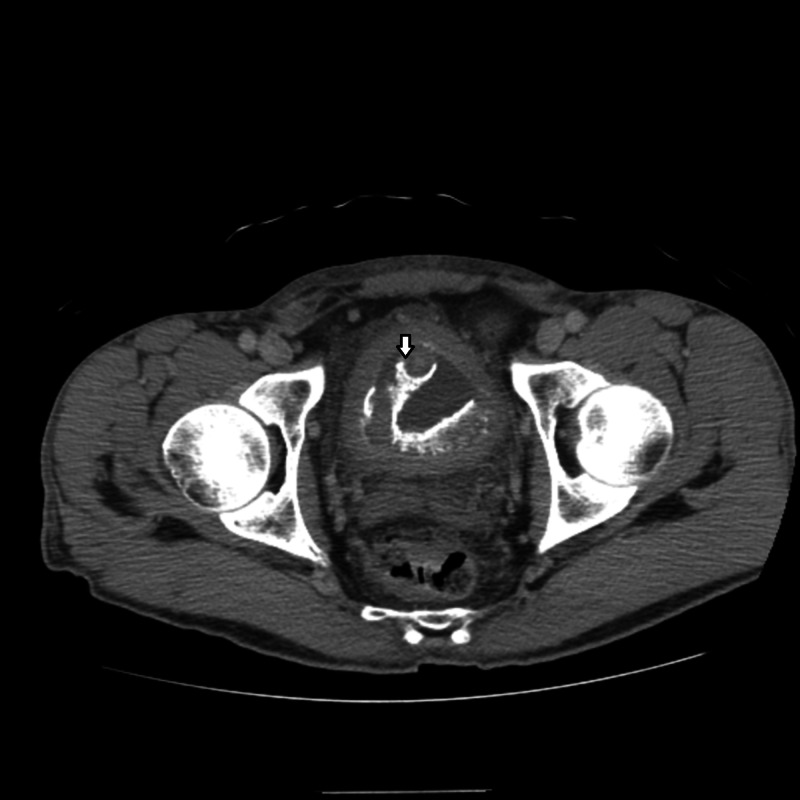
CT urogram demonstrating coiled tube like structure in the urinary bladder.
